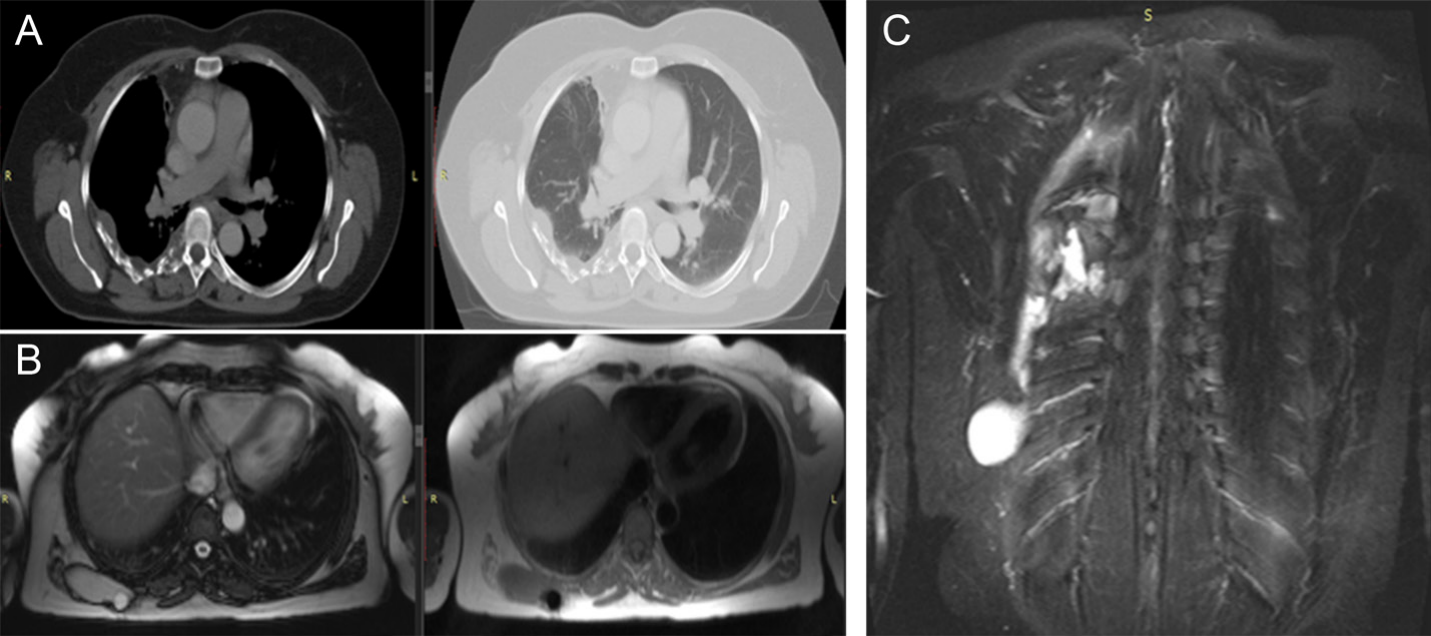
Thoracic computed tomography (A) scan showing intramedullary lytic and enlarging masses posterior to the fourth, fifth, and sixth ribs on the right. A hydatid cyst is seen in the right latissumus dorsi muscle on the T1- and T2-weighted thorax magnetic resonance image (MRI) (B) of the same patient. Demonstration of cysts in the same patient on coronal T2-weighted thorax MRI (C).
